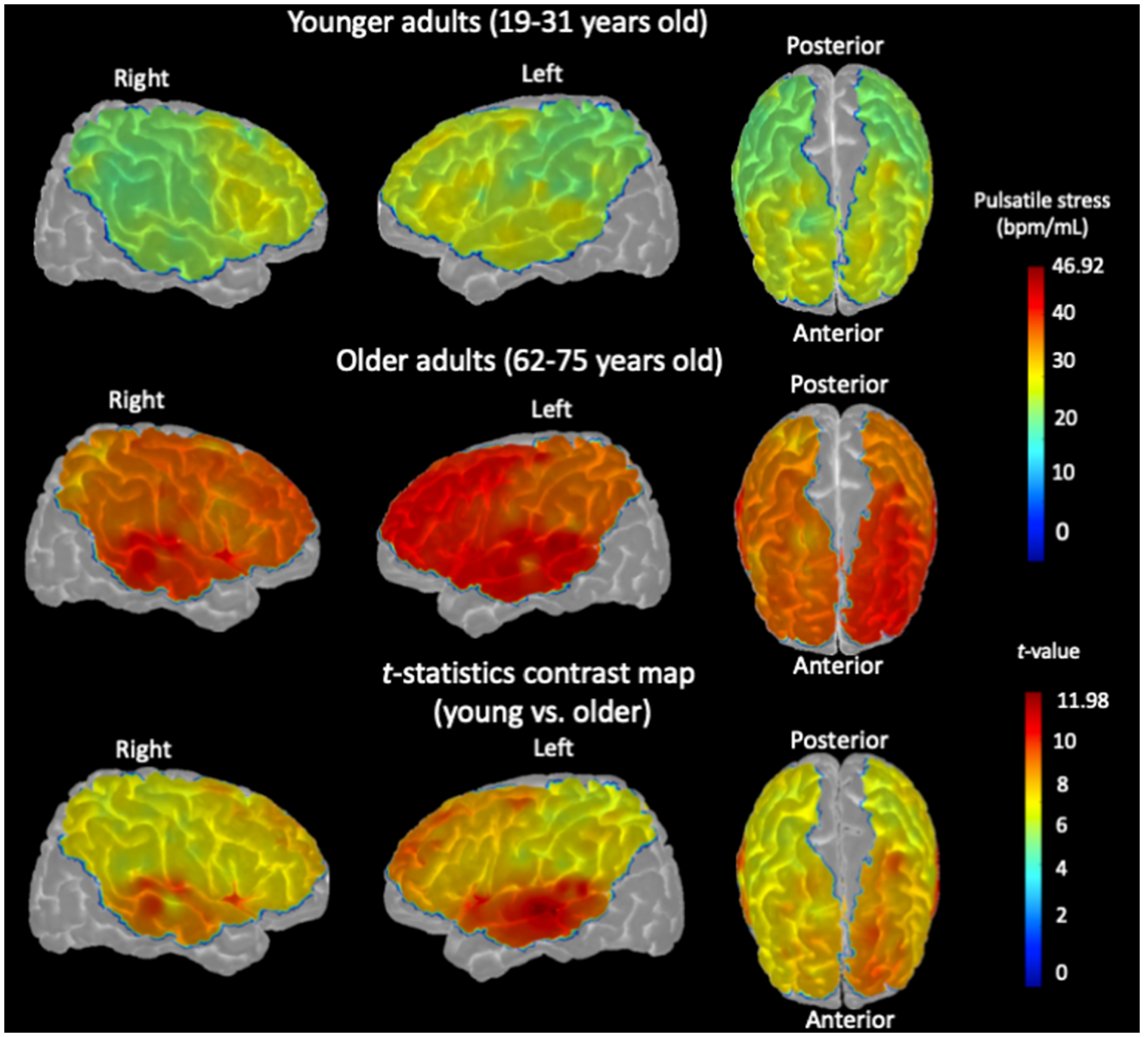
Channel-wise cerebral pulsatility index, averaged across all participants, projected onto the cortex of the Colin27 template. For each individual, the product of cerebral pulse amplitude and heart rate for each NIRS channel in each hemisphere was normalized by dividing by the hemispheric BV pulsatility index measured by PC-MRI. (Top row): Cerebral pulsatility index map for younger adults. (Middle row): Cerebral pulsatility index map for older adults. Higher values on the map (red regions) indicate greater pulsatility, whereas lower values (green regions) indicate a ﻿lower pulsatility index. (Bottom row): *t*-statistic contrast map of the channel-wise comparison between older adults and younger controls, with *p* < 0.05, FDR-corrected.
